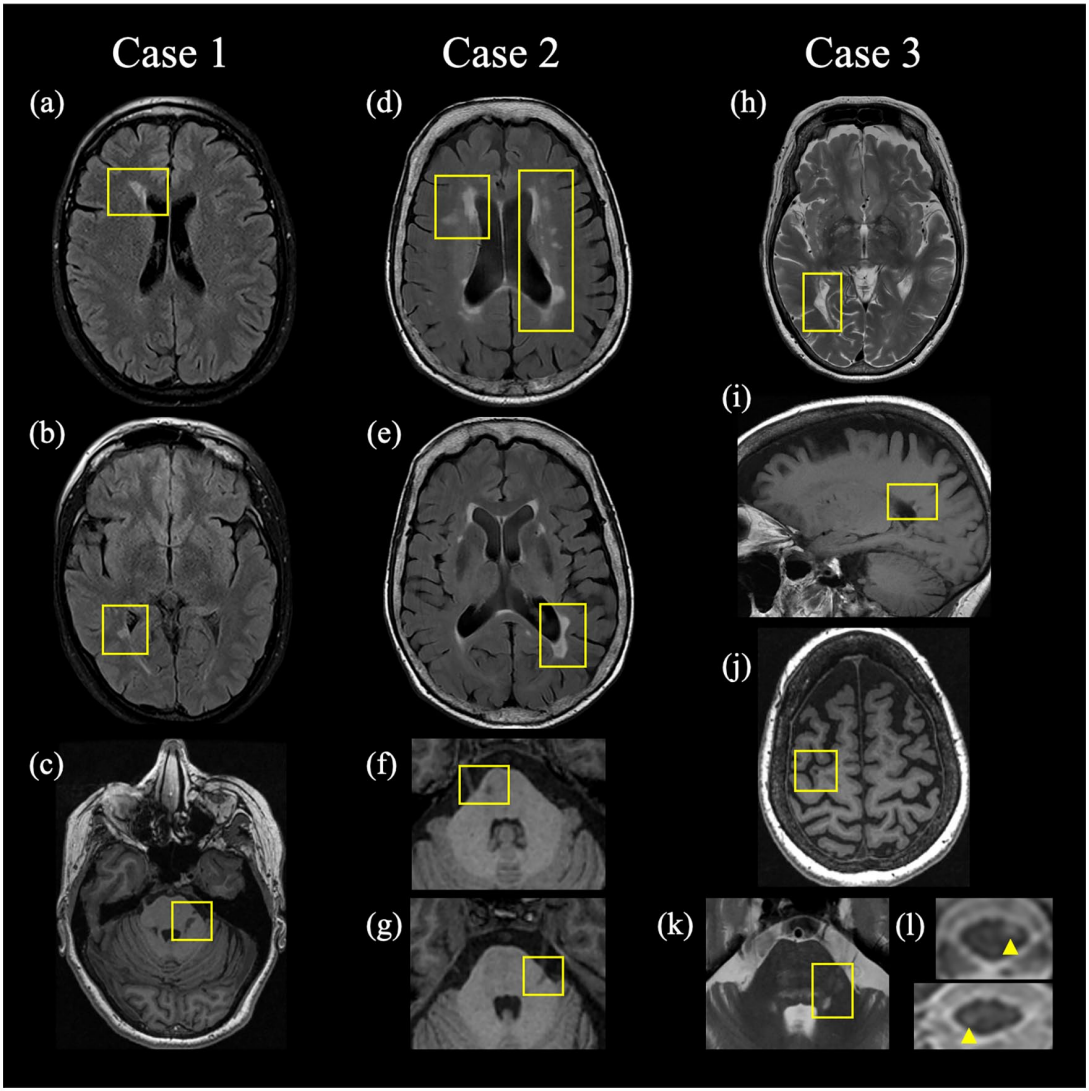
Magnetic resonance imaging sequences demonstrating demyelinating lesions characteristic of MS. Left column: axial T2-FLAIR MRI demonstrating periventricular lesions (a, b) and axial T1-weighted FSPGR MRI demonstrating a left-sided pontine lesion presumed responsible for TN (c) in Case 1. Middle column: axial T2-FLAIR MRI demonstrating periventricular lesions (d, e) and axial T1-weighted FSPGR MRI demonstrating infratentorial lesions (f, g), with the left-sided lesion (g) presumed responsible for TN in Case 2. Right column: axial T2-weighted and sagittal T1-FLAIR MRI demonstrating periventricular lesions (h, i), axial T1-weighted FSPGR MRI demonstrating a juxtacortical lesion (j), and axial T2-weighted MRI demonstrating the pontine lesion presumed responsible for TN (k) and spinal cord (l) lesions in Case 3. FLAIR: fluid-attenuated inversion recovery; FSPGR: fast spoiled gradient-echo.
